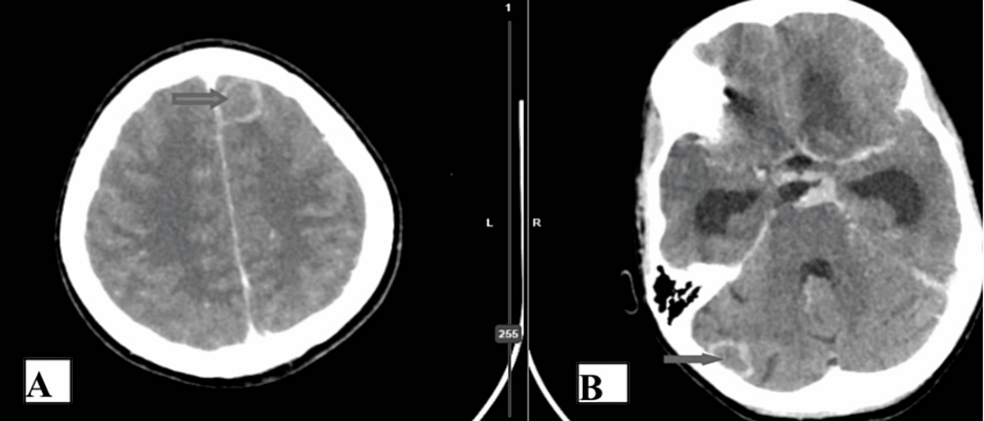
Preoperative CT brain scan (contrast study, axial section) of a patient showing tuberculomas in the left frontal region and right cerebellum.
